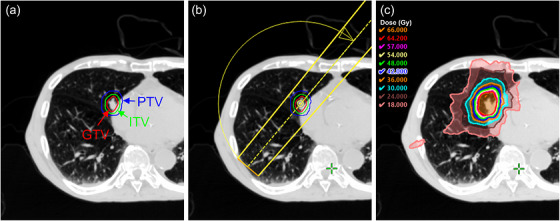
Overview of a representative clinical case (Patient Case 1) used for the dosimetric impact assessment. (a) Axial CT image showing the delineation of the GTV, ITV, and PTV. (b) Beam arrangement for the VMAT plan utilizing a partial arc. (c) Resulting dose distribution.
